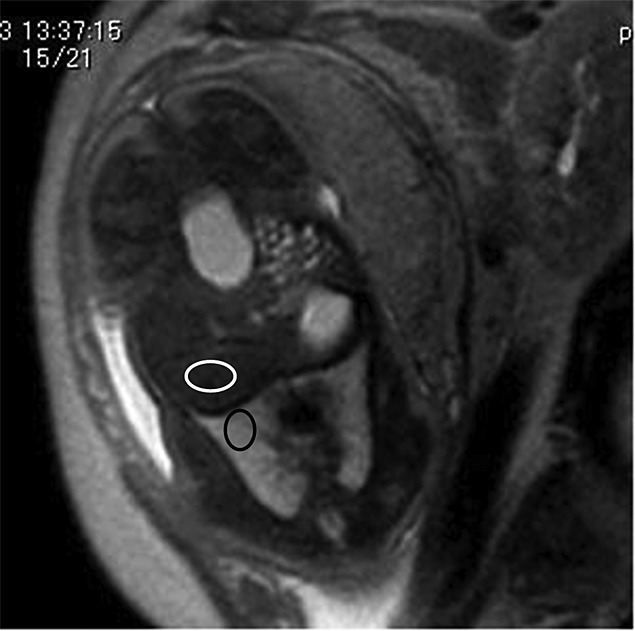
Half-Fourier acquisition single-shot turbo spin-echo magnetic resonance image of fetus at the 37^th^ week of gestation shows regions of interest in both lung (black ellipse) and liver (white ellipse). Chosen areas are homogeneous and free of vascular and tracheal structure
